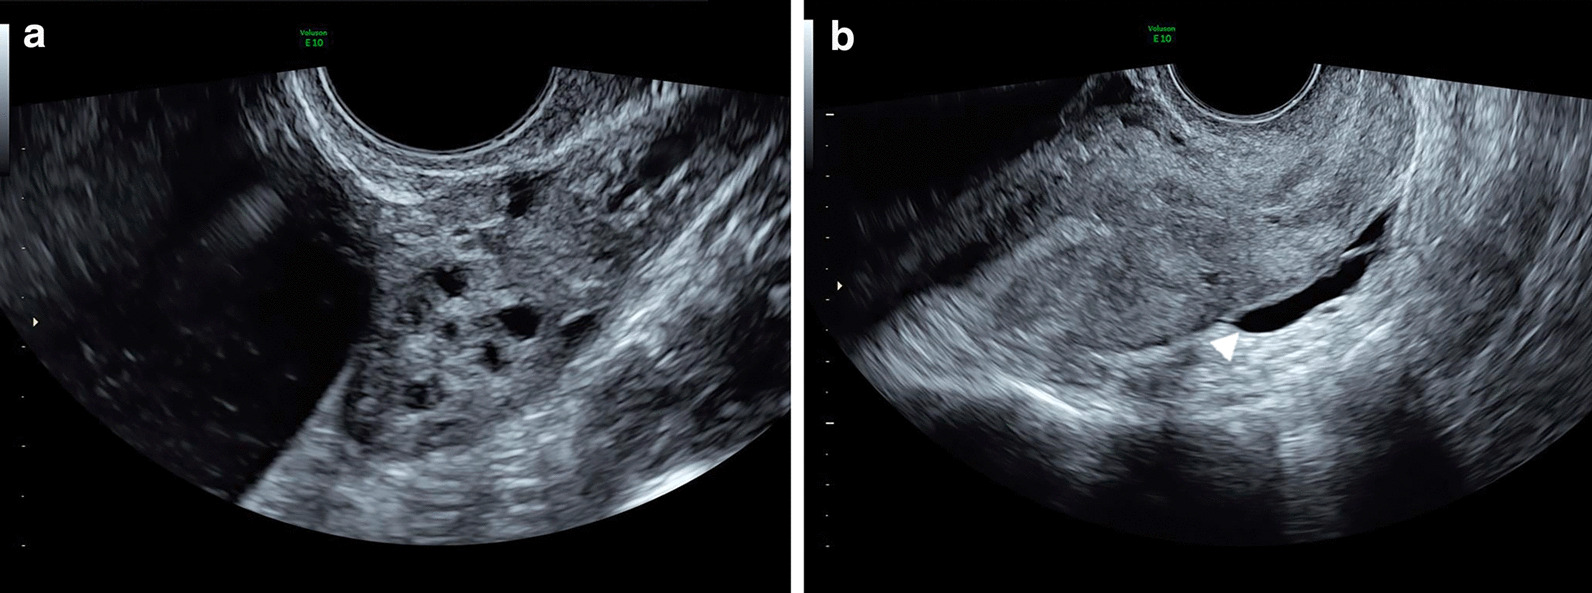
Grayscale transvaginal ultrasonography: left ovary (a) and uterus (b). Fluid in the pouch of Douglas (arrowhead)
